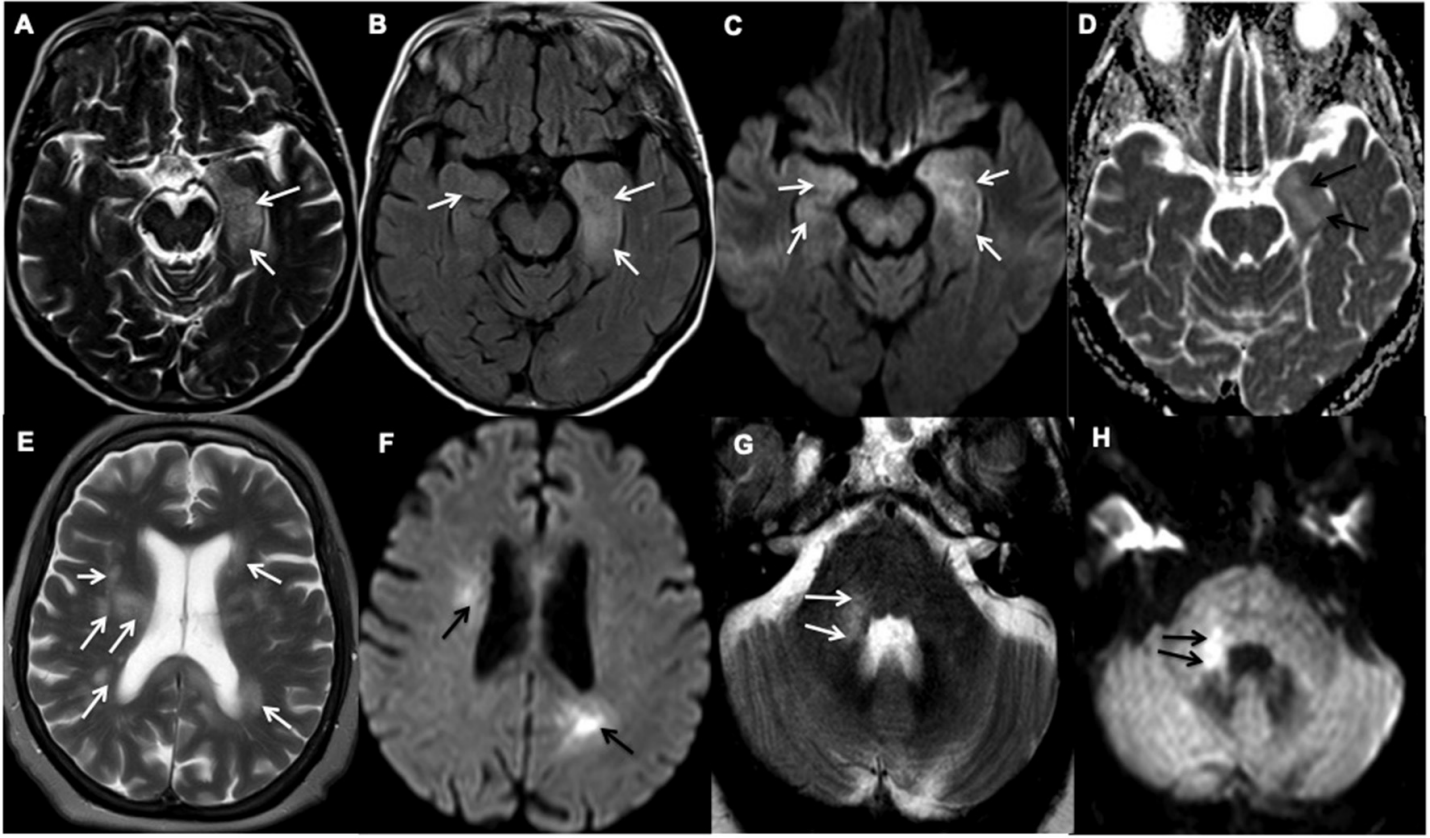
Representative examples of inflammatory encephalopathy. (A–D) Axial MR images of Patient 21 demonstrate limbic encephalitis. (A) T2-weighted and (B) Fluid attenuated inversion recovery (FLAIR) imaging demonstrate hyperintensity in both medial temporal lobes, but predominantly in the left amygdala and hippocampus (white arrows), (C) Diffusion-weighted imaging (white arrows) and (D) Apparent diffusion coefficient (ADC) show corresponding partial diffusion restriction (black arrows). (E–H) Axial MR images of Patient 27 demonstrate acute demyelinating encephalomyelitis. (E) T2-weighted imaging demonstrates patchy and asymmetric white matter hyperintensities within the periventricular and subcortical regions and (G) right middle cerebellar peduncle (white arrows). (F,H) Diffusion-weighted imaging shows corresponding high diffusion signal (black arrows).
